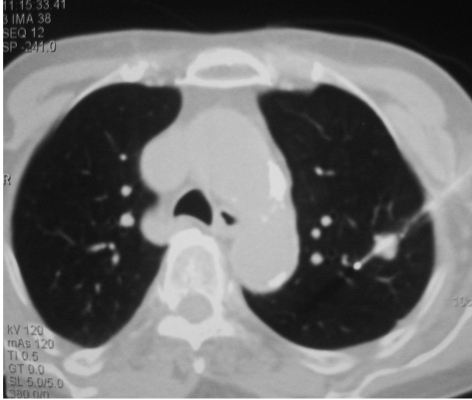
RF electrode inserted into lesion under CT fluoroscopic guidance. Note that the tip of the electrode has gone beyond the far margin of the tumour nodule to ensure that a good rim of normal lung tissue is also ablated.
